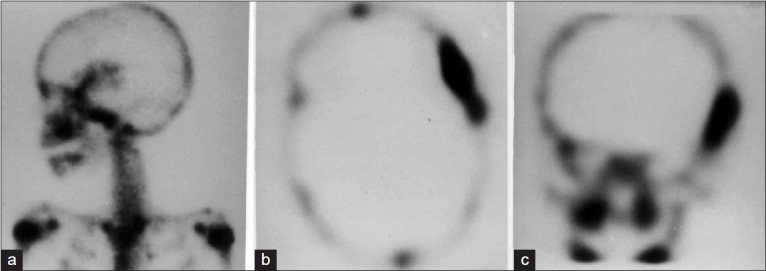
Left lateral view of the skull (a) of the Tc99m MDP static planar bone scan showing an oval area of increased tracer concentration in the left temporal region. Transverse section (b) and Sagittal view (c) of bone the SPECT of the skull showing the extent of the increased tracer concentration in the left temporal region involving full thickness of the bone
